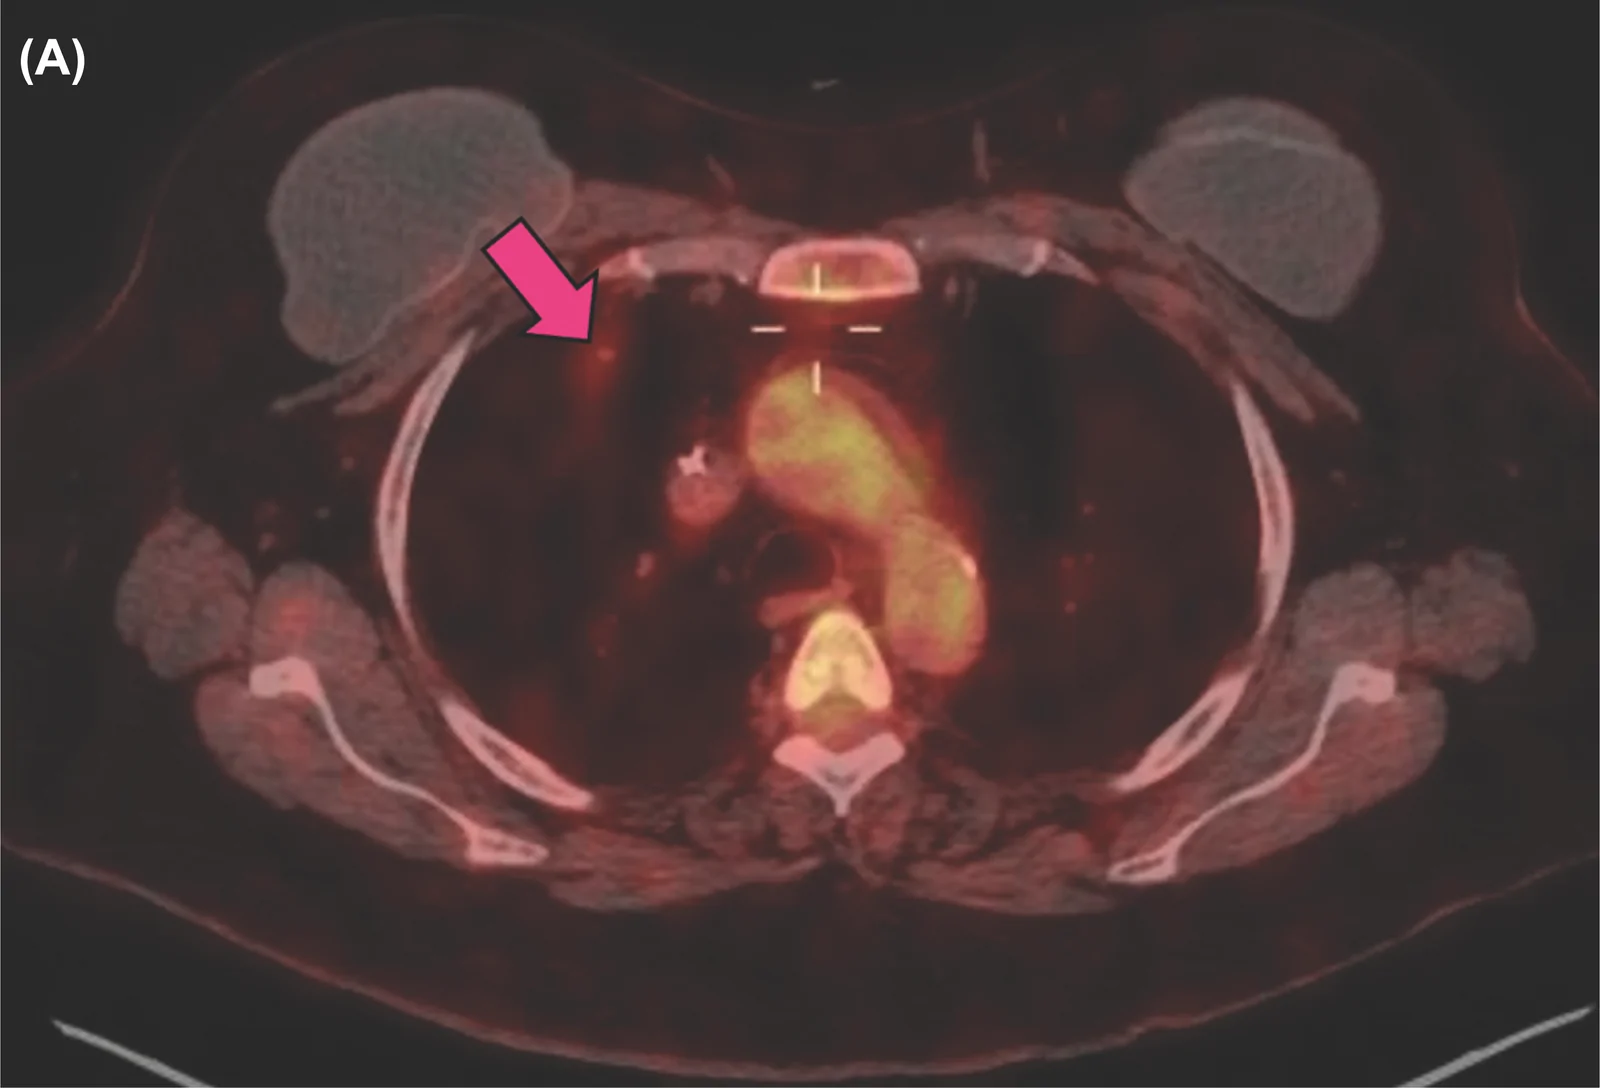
18F-FDG PET/CT (~3-month follow-up). Decreased size and metabolic activity of the previously noted right upper-lobe pulmonary nodule (pink arrow), consistent with interval treatment response. Abbreviations: FDG, fluorodeoxyglucose; PET/CT, positron emission tomography/computed tomography; SUVmax, maximum standardized uptake value.
